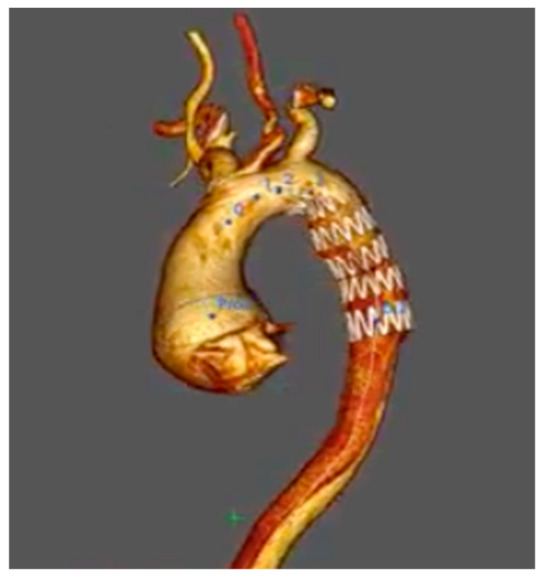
Pre-operative computed tomography (CT) aortogram. Figure legend: Pre-operative computed tomography aortogram demonstrating obstruction of the TEVAR lumen.
